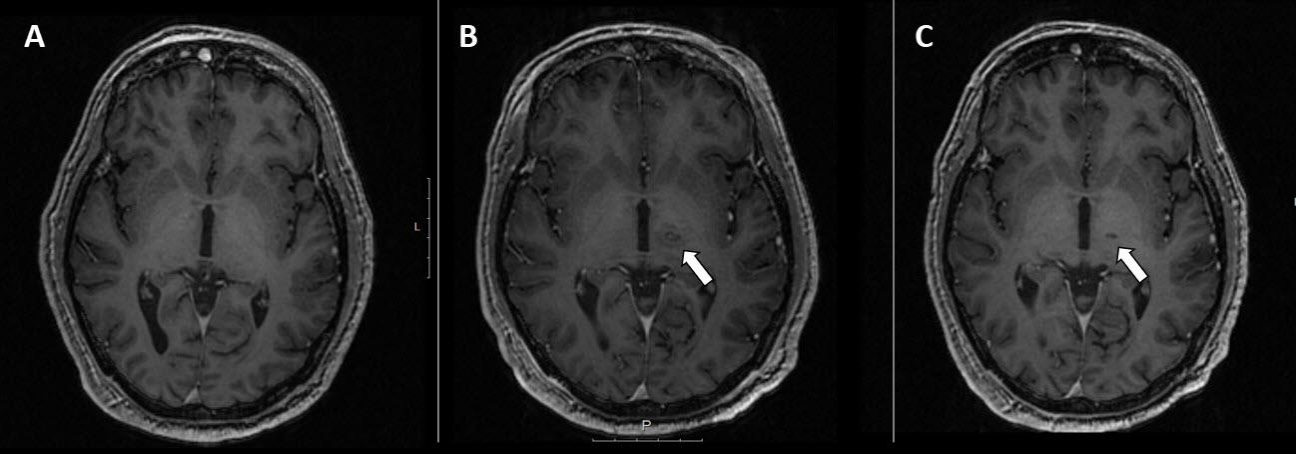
Magnetic Resonance-guided Focused Ultrasound (MRGFUS) Axial T1-weighted post-contrast MR images (A) before MR-guided focused ultrasound (MRGFUS) treatment. B: One day after MRGFUS to the right basal ganglia, showing the ablated area with surrounding edema (arrow). C: One year after treatment, showing a small area of encephalomalacia (arrow).
